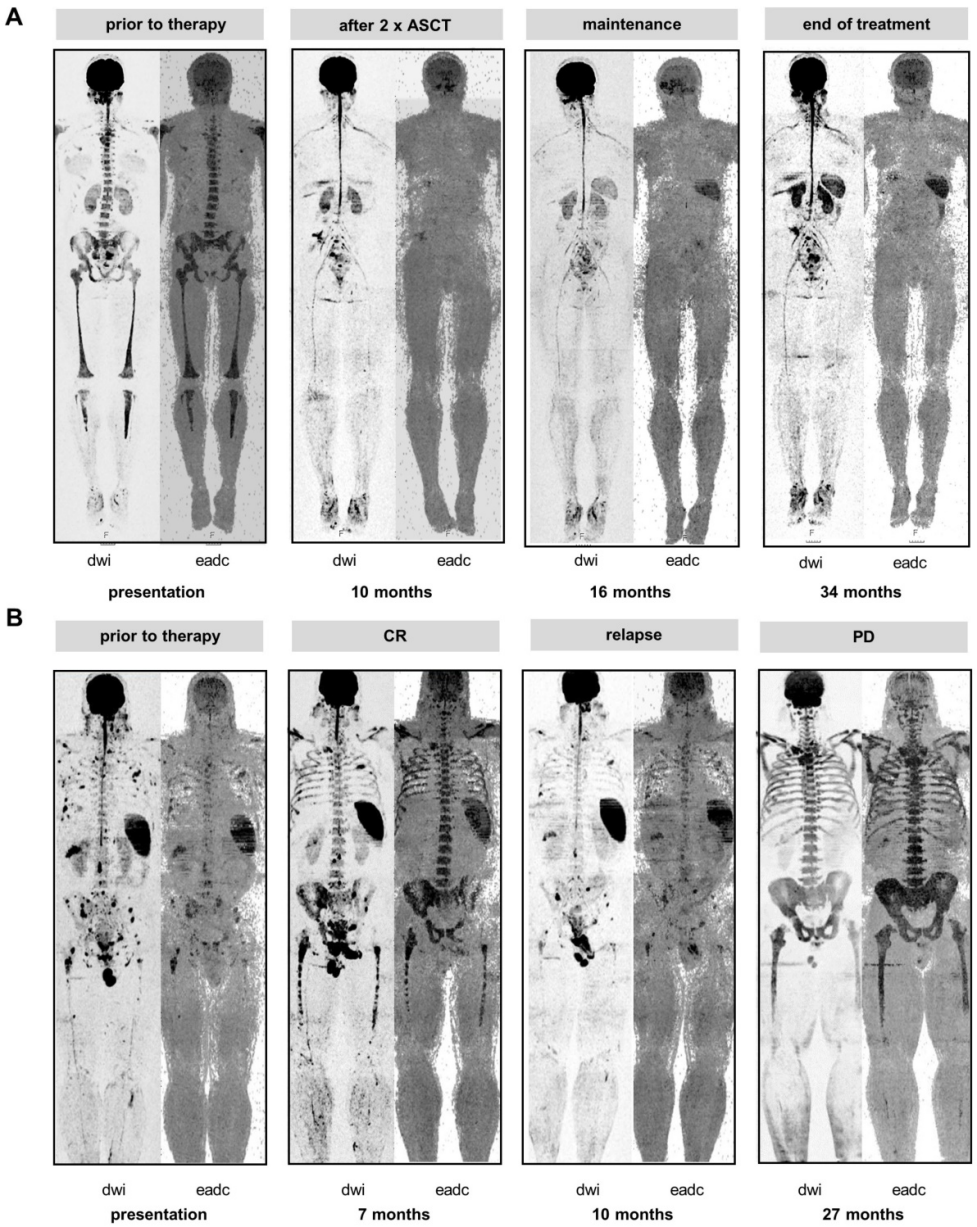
The spleen signal during therapy. A Baseline and follow-up inverted greyscale b800 DW-MRI images and EADC maps are shown for one patient who initially presented w/o spleen signal but experienced re-appearance of the signal during therapy. B Loss of spleen signal during the course of the disease. The patient relapsed with multiple small focal lesions. Subsequently, the patient experienced progressive disease (PD) and developed a severe diffuse background pattern with concomitant loss of the spleen signal.
